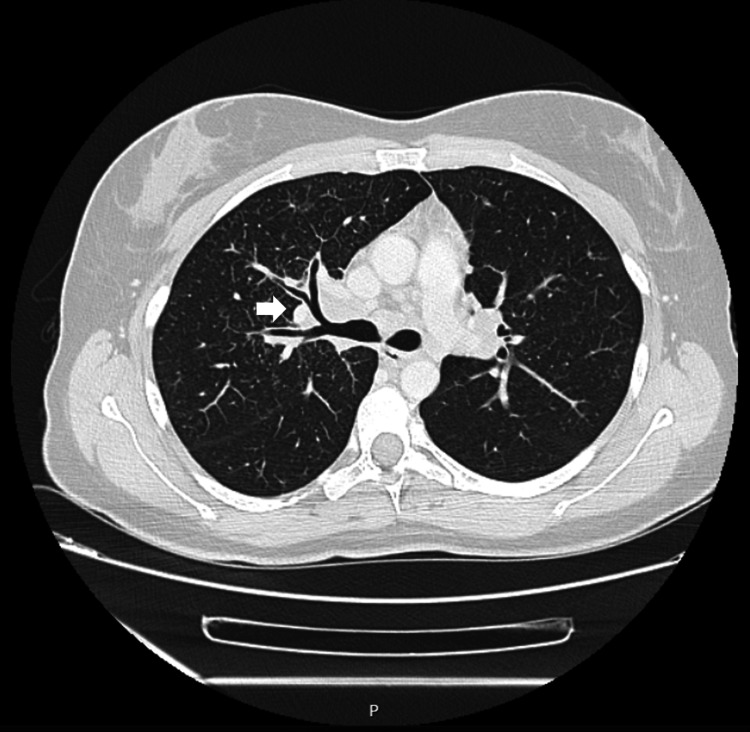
Second chest computed tomography scan showing the progression of the hilar lymphadenopathy clusters marked with an arrow (July 18, 2024).
